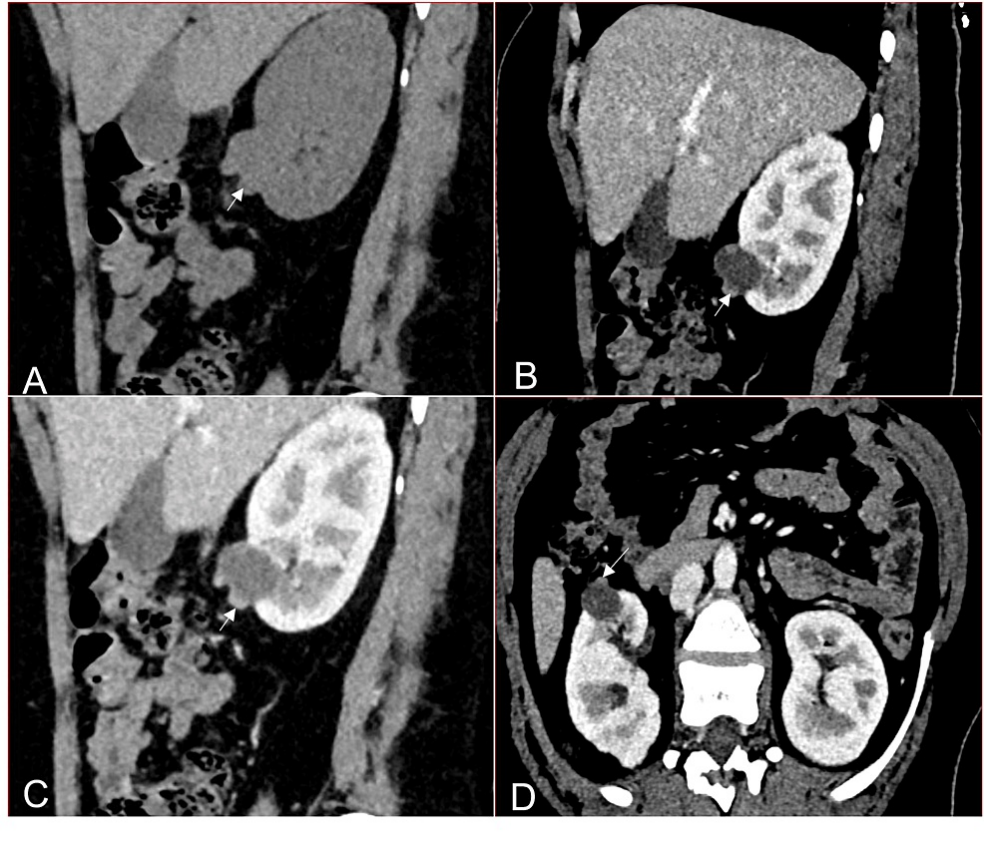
A 60-year-old male with a right renal lesion Reformatted oblique NCCT (A), corticomedullary phase (B), and nephrographic phase (C, D) images show an exophytic cyst in the right kidney lower pole. The cyst is hypodense with an eccentric enhancing nodule <3 mm in thickness (white arrows). This has been categorized as a Bosniak III lesion. The nodule shows enhancement in the corticomedullary phase and nephrographic phase. NCCT: non-contrast-enhanced CT
